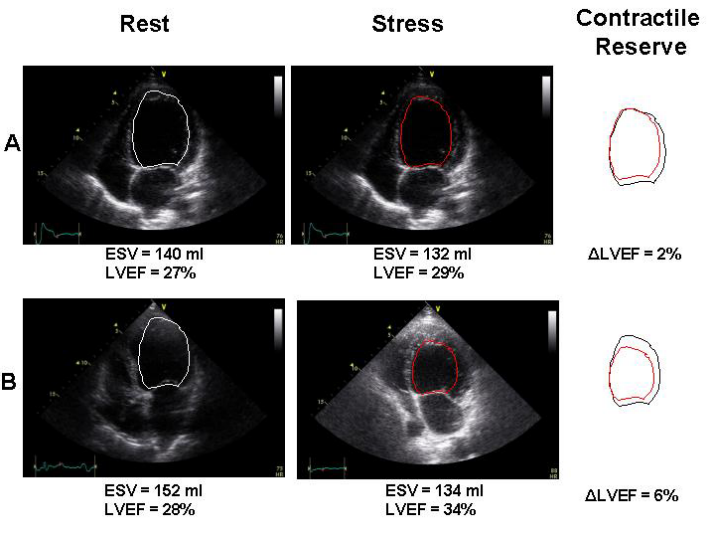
Echocardiographic apical four-chamber images (end-systolic frames) from two patients with and without contractile reserve.
